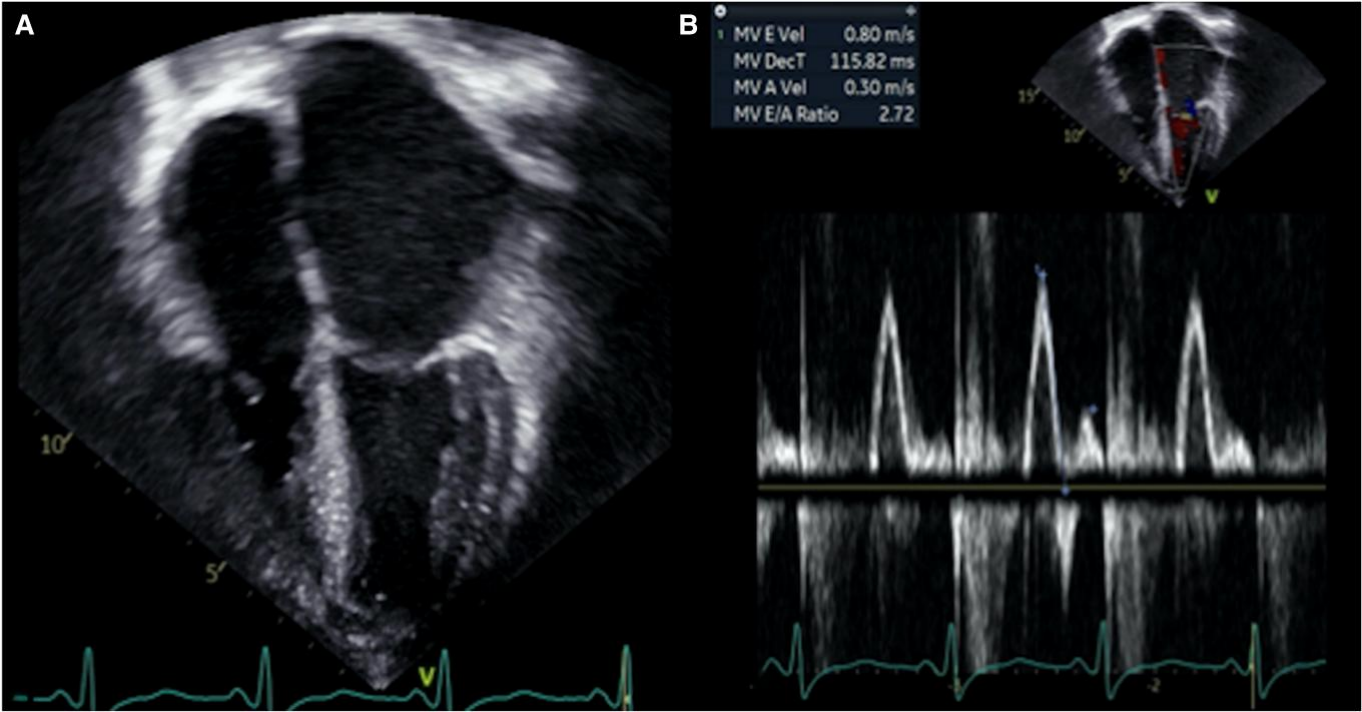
(*A*) Apical four-chamber echocardiographic view showing severe biatrial enlargement, with a markedly increased left atrial volume index of 68.7 mL/m^2^. The left ventricular chamber size remains within normal limits, highlighting the disproportionate degree of atrial dilatation relative to ventricular dimensions. (*B*) Pulsed-wave Doppler of mitral inflow showing an elevated *E*/*A* ratio (2.72) with a shortened deceleration time (116 ms) according to age values, findings consistent with chronically elevated left ventricular filling pressures and restrictive physiology.
